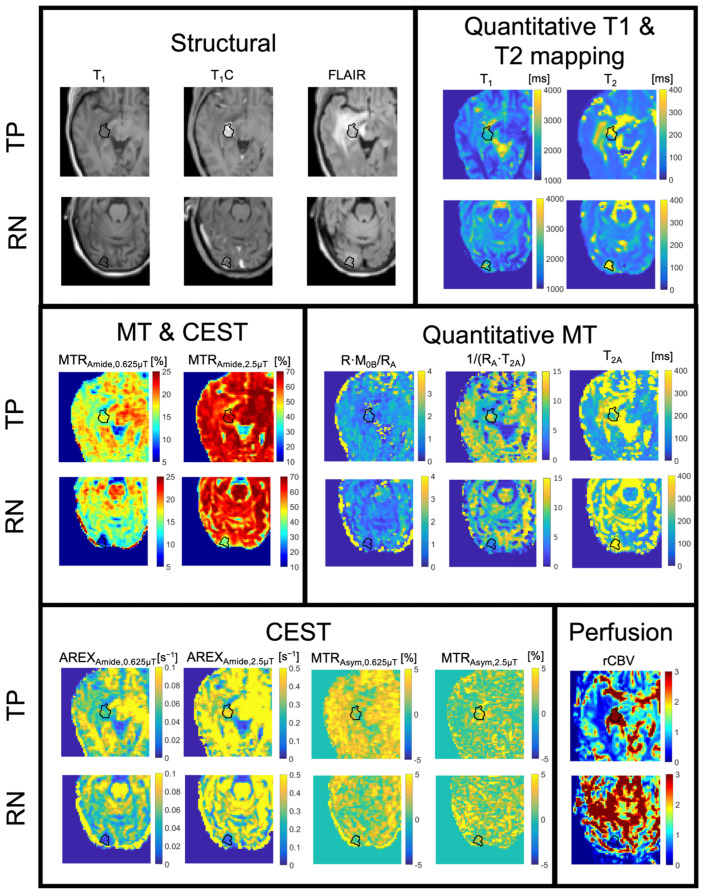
Parameter maps for one example of tumor progression (TP) compared to one example of radiation necrosis (RN). The panels divide images by contrast: structural images (pre- and post-contrast T_1_-weighted and FLAIR), quantitative T_1_ and T_2_ mapping, magnetization transfer and chemical exchange saturation transfer ratios (MT & CEST), and quantitative MT, CEST, and DSC perfusion. Within each panel, the top row shows the case of tumor progression and the bottom row the case of radiation necrosis. The black region-of-interest overlays show the whole-tumor contours for each patient. Patients had prior SRS with radiation doses of 16 Gy in one fraction (TP case) and 25 Gy in five fractions (RN case). This figure is adapted from Chan et al. under a Creative Commons License [143].
